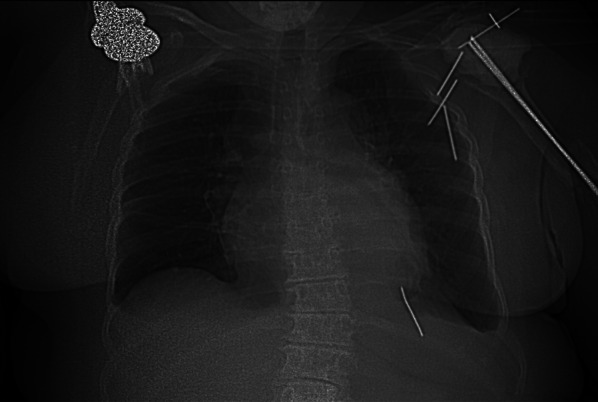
Scout view of preoperative thoracic CT scan
